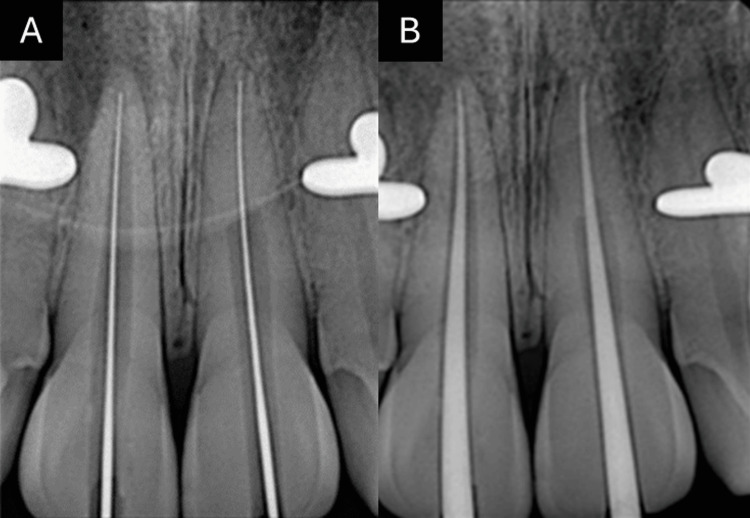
(A) Working length radiograph and (B) master cone radiograph
